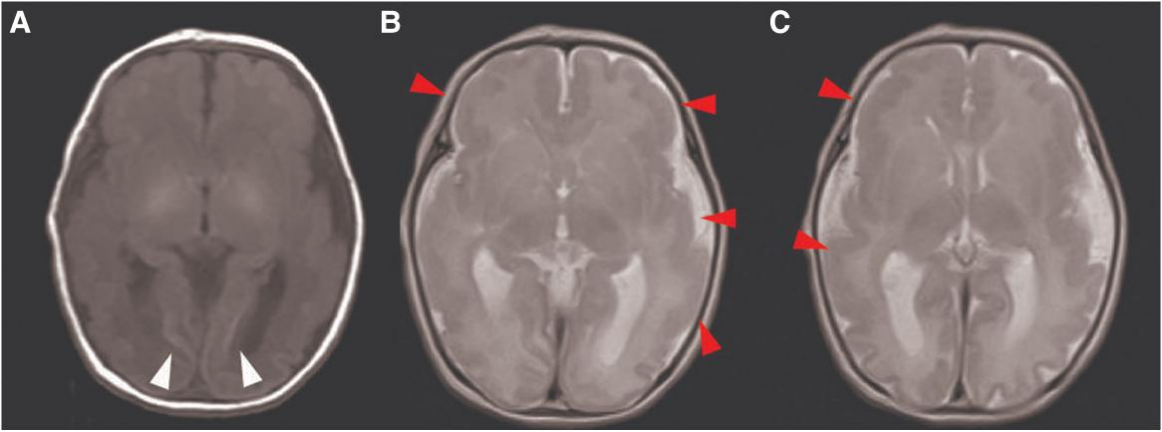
Brain magnetic resonance imaging scan performed on the day of birth. (*A*) T1-weighted images show high signal intensity at the corticomedullary border, indicating calcification (white arrow). (*B* and *C*) T2-weighted axial images depict polymicrogyria with cortical thickening extending from the parietal to temporal areas, predominantly in the frontal areas on both sides (red arrow).
